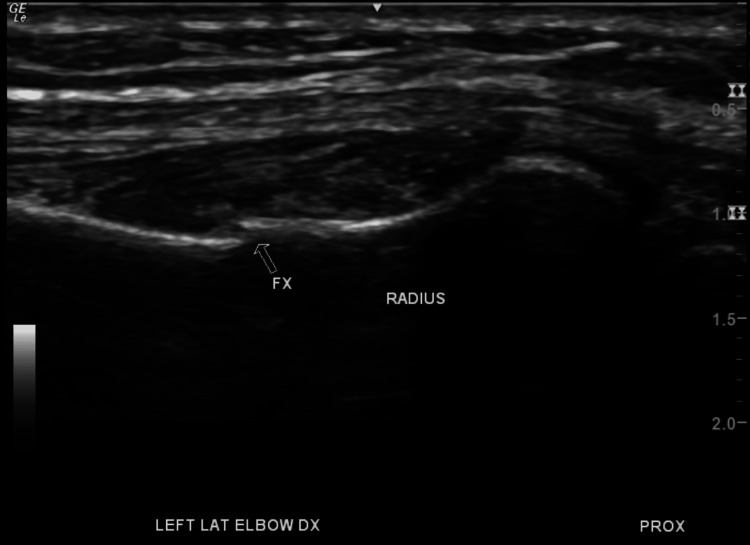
Forearm ultrasound with arrow showing the location of the fracture Ultrasound image of the patient’s left forearm. The figure shows a 1.5 mm cortical offset, indicating a possible fracture along the radial shaft distal to the radial head.
